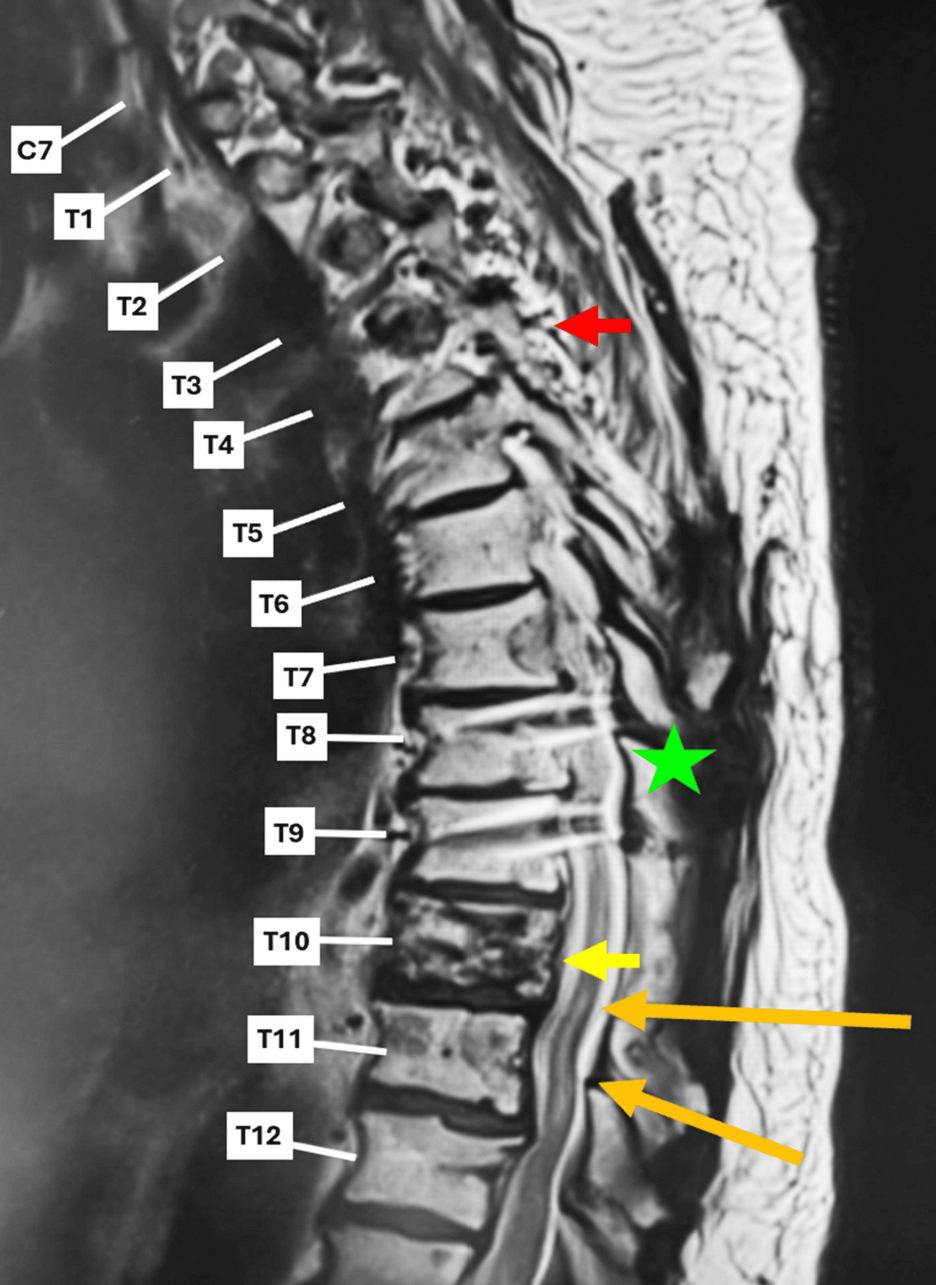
Thoracic spine MRI taken on ED admission shows postoperative changes T-spine MRI showing postoperative changes from the laminectomy at T10 (orange arrows), changes from transpedicular fusion at T8-12 (green star), T2 hyperintensity in the spinal cord from T3 (red arrow) to T7, and multiple metastatic deposits present in the thoracic spine, especially at T10 (yellow arrow).
